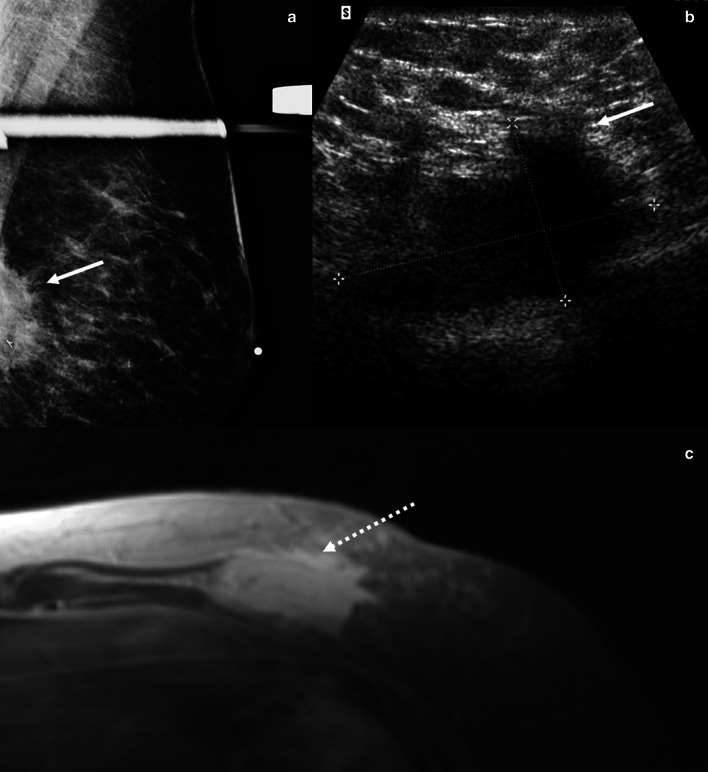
Desmoid fibromatosis. A 47-year-old woman presented with a palpable mass. Lateral spot compression mammogram (a) shows an irregular, high-density mass with spiculated margins adjacent to the chest wall (arrow). Grayscale ultrasound (b) shows a corresponding irregular, hypoechoic anti-parallel mass with indistinct margins. T1-weighted post-contrast fat-saturated MRI of the chest (c) shows invasion of the pectoralis major muscle by this irregular enahancing mass (dotted arrow). Core needle biopsy showed desmoid fibromatosis. The patient underwent surgical excision
